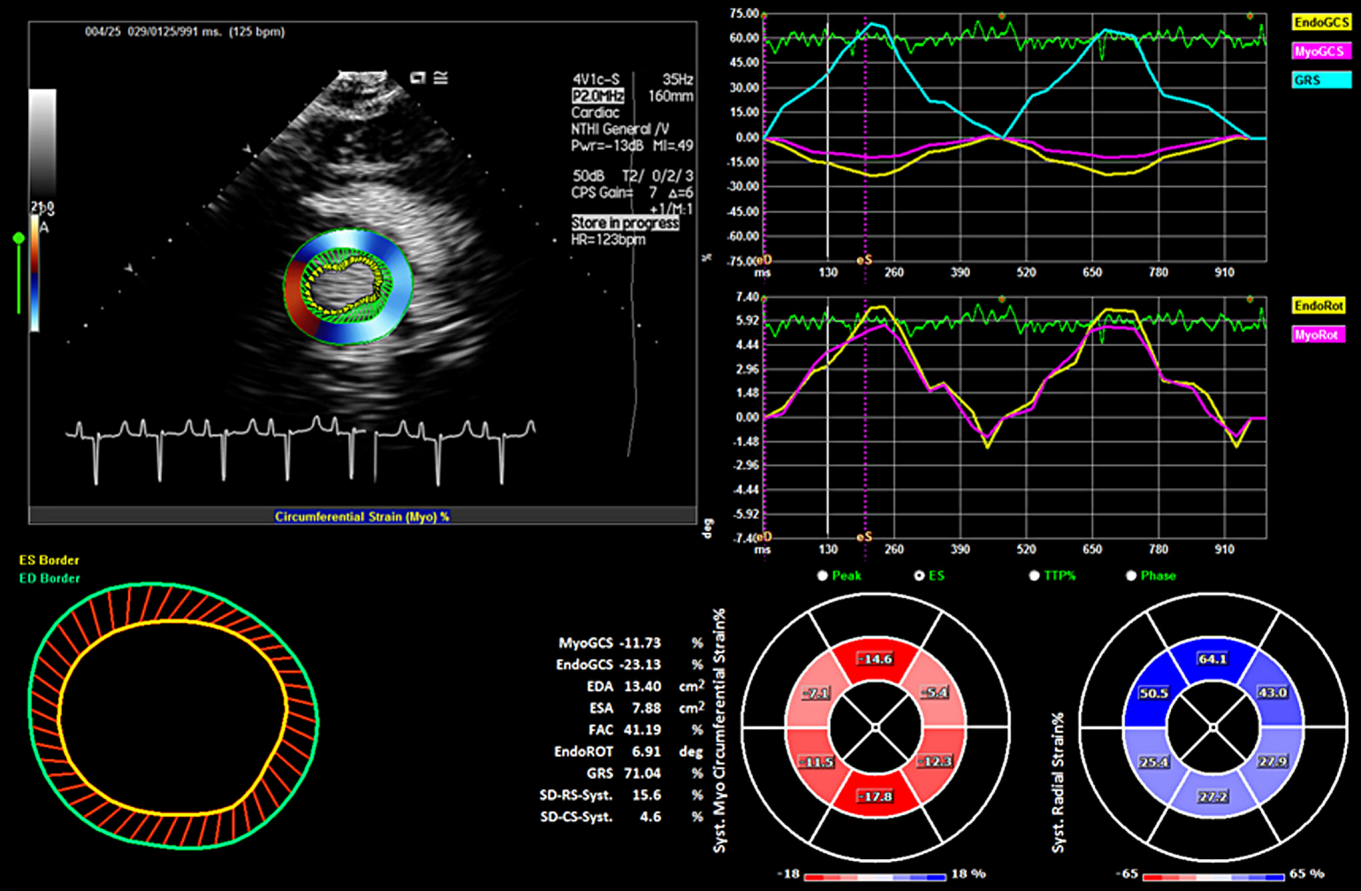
Analytical interface for contrast-enhanced imaging in the parasternal short axis view, showing the EDA, ESA, FAC results (lower left panel) and the EGCS, MGCS, GRS and ER (upper right panels). Reprinted from *Heart, Lung and Circulation*; vol 27, Supplement 2; Platts D, Shiino K, Chan J, Burstow D, Scalia G & Fraser J; Comparison of unenhanced and contrast-enhanced echocardiographic assessment of myocardial function and mechanics during veno-venous extracorporeal membrane oxygenation; page S228; Copyright (2018), with permission from Elsevier. Video 2 shows the corresponding clip.
